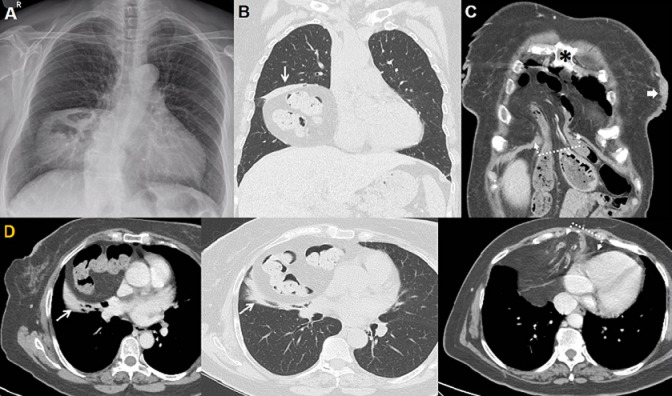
A) chest X-ray: well-defined heterogenous mass with presence of air adjacent to right heart aspect, between diaphragm and minor (horizontal) fissure; scoliosis of the spine is also noted; B, C) CT reconstruction in coronal plane (lung and mediastinal window) revealed bowel loops herniated from foramen of Morgagni (note the para-retrosternal position-black star- of bowel loops which insert from a diaphragmatic foramen -dot line); focal thickening of the left breast skin (arrow), consisted with the known cancer; D) the same findings in axial views in lung (middle view) and in mediastinal window; thin arrows (including B view): compressive atelectasis
